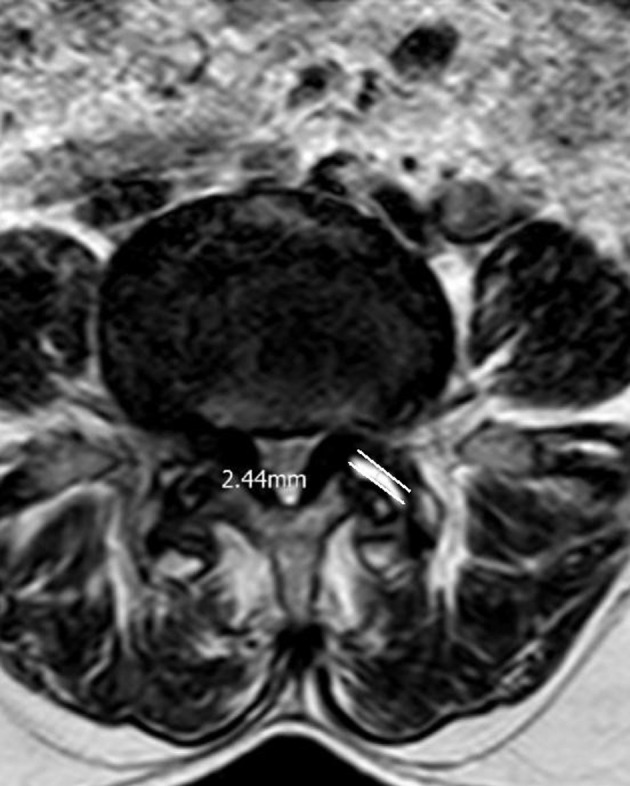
Measurement of small joint effusion. Measurement of facet joint effusion width on axial T2-weighted MRI. The axial slice showing the largest facet joint space was selected and the maximal anteroposterior distance between opposing articular surfaces (white line/arrow) was measured perpendicular to the joint plane. The measured distance is reported as the maximum facet joint effusion width (mm). MRI: magnetic resonance imaging.
